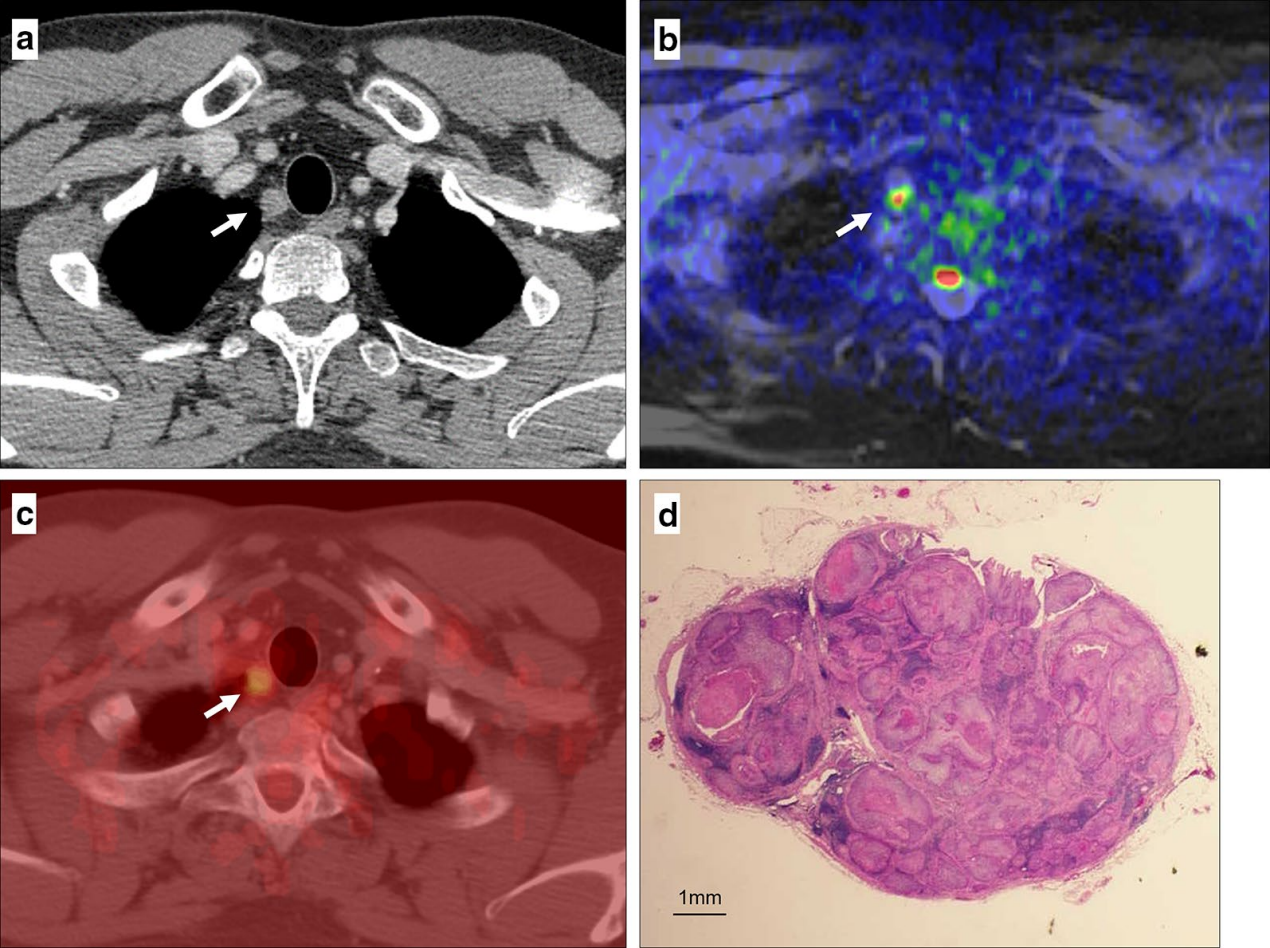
True positive lymph node of 10 mm in size by DWI and PET (arrow). a Thoracic paratracheal lymph node was detected on contrast-enhanced CT as metastasis. It was hyperintense on DWI (b) and hyperaccumulate on PET (c). d The node was identified as metastatic with 100% of intranodal cancer nest
